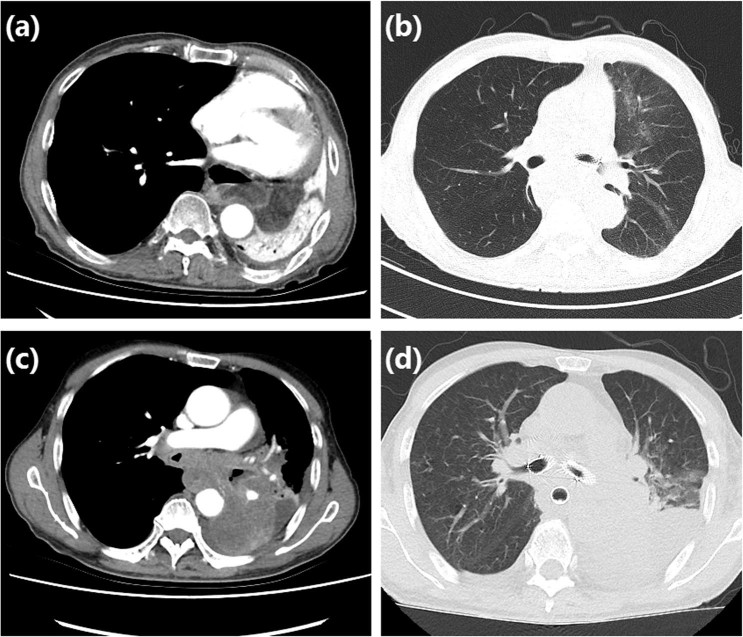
Chest CT examination. (a) The lung tissue of atelectasis was obviously enhanced before operation, and completely ventilated after stent placement (b). (c) The enhancement of left inferior lung tissue was poor before operation, (d) and the lung was not ventilated after stent.
